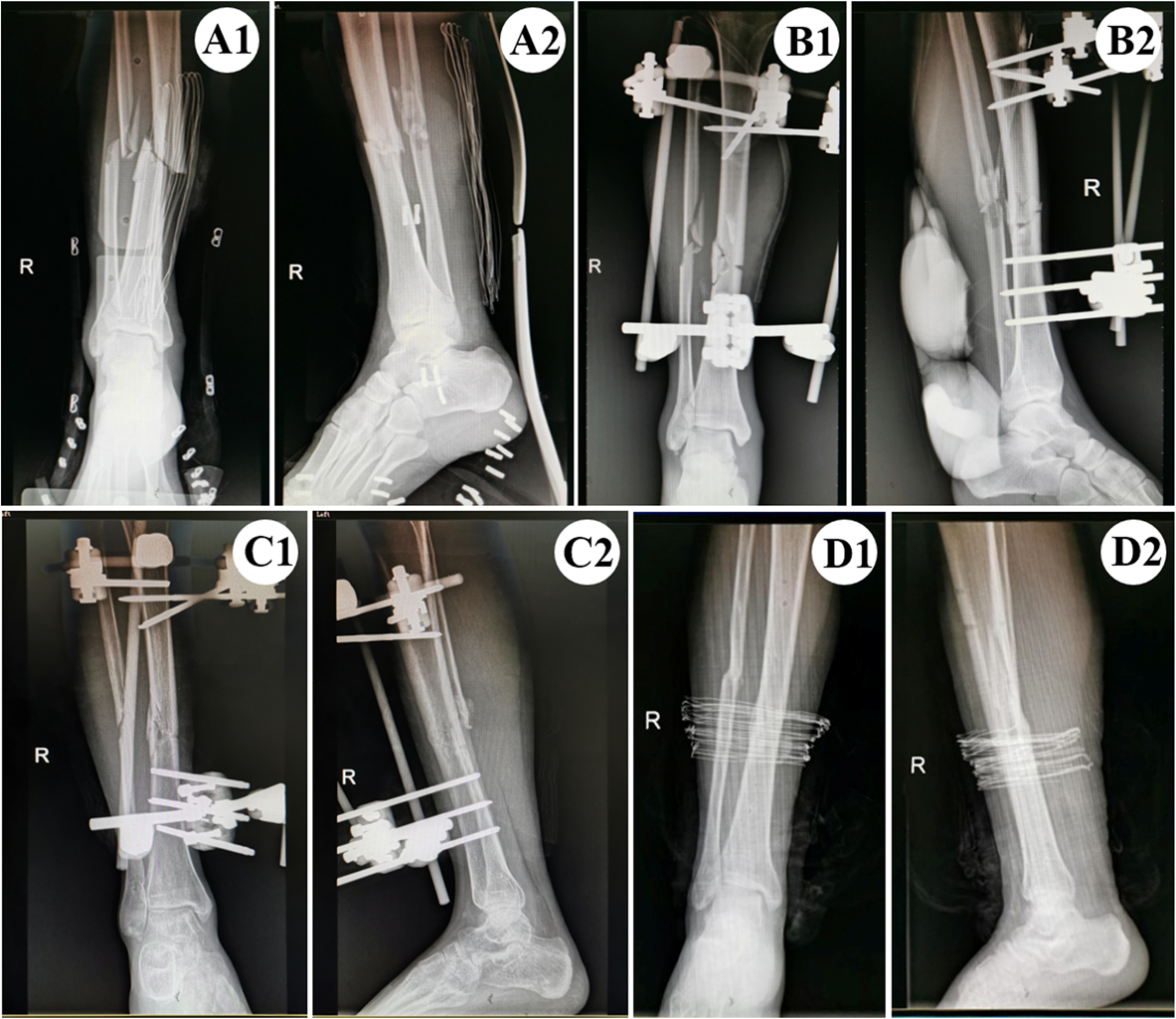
A1-A2 Anteroposterior and lateral radiograph of distal tibiofibular fractures before operation. B1-B2 Bedside X-ray examination 2 days after operation (anteroposterior and lateral views). C1-C2 The fracture showed radiological signs of healing 7 months after operation (anteroposterior and lateral views). D1-D2 The external fixator was removed completely 10 months after operation (anteroposterior and lateral views)
